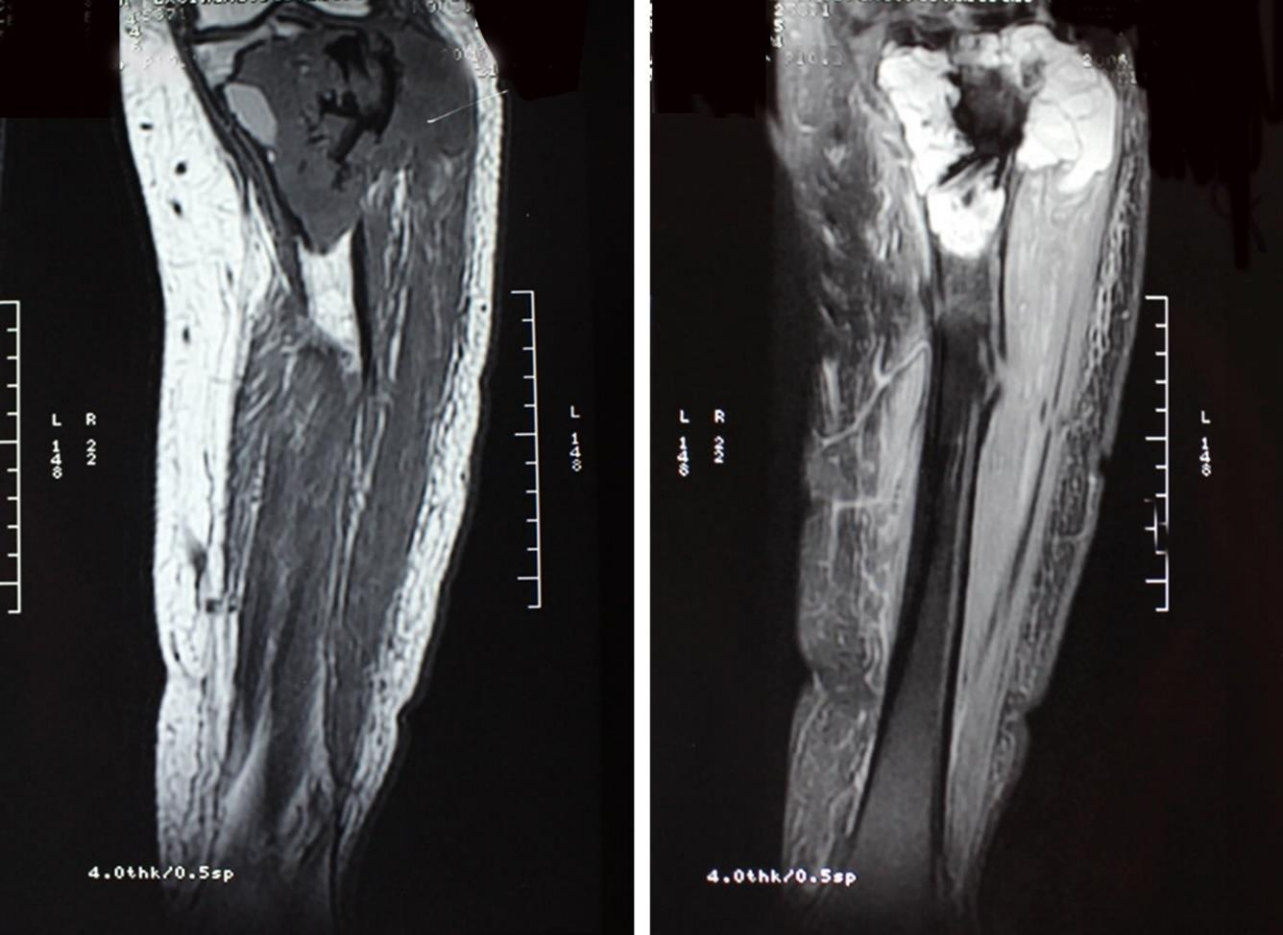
Magnetic Resonance Imaging at recurrence (6 years later), showed a destructive lesion associated with the soft tissue component and signal void center due to prior treatment with bone cement
